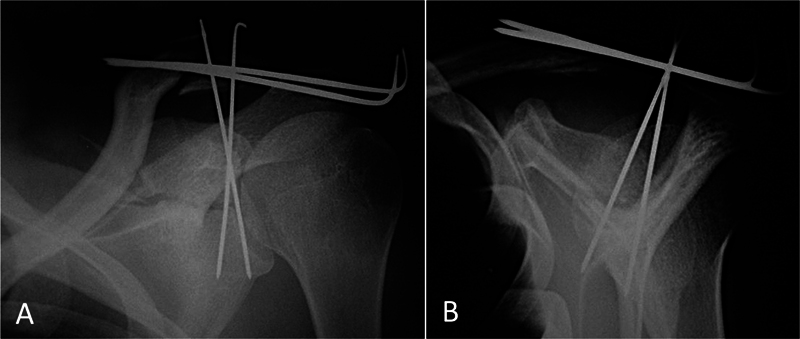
Radiographs of the left shoulder demonstrating immediate postoperative follow-up in anteroposterior (A) and lateral (B) views after percutaneous fixation of the scapula and distal clavicle fractures with Kirschner wires. Source: authors' archive.
